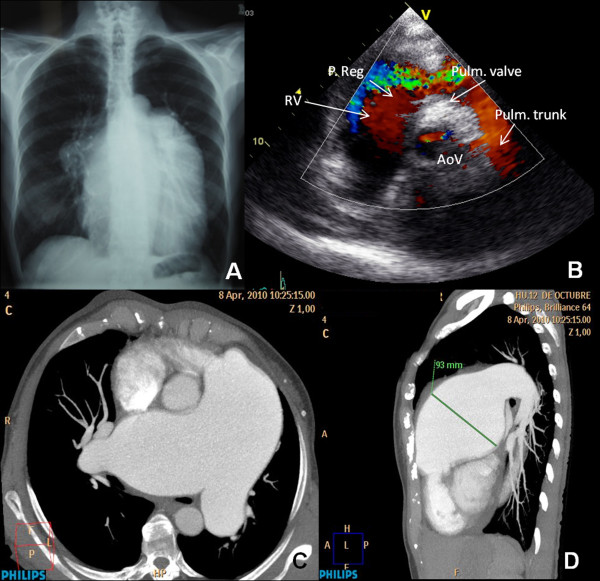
Imaging exams displaying the pulmonary aneurysm and associated features. A) Chest radiograph (posteroanterior view) shows cardiomegaly, dilated main pulmonary artery and right pulmonary artery. B) Ecocardiography: paraesternal view, at great vessels level. Color-Doppler shows severe pulmonary regurgitation. C) Multislice contrast computed tomographic scan of the thorax on axial projection shows a close contact of the main pulmonary artery to the chest wall, dilated main pulmonary artery and both of its branches which have a fusiform morphology. D) On sagital projection the widest diameter of the aneurysm can be measured.
